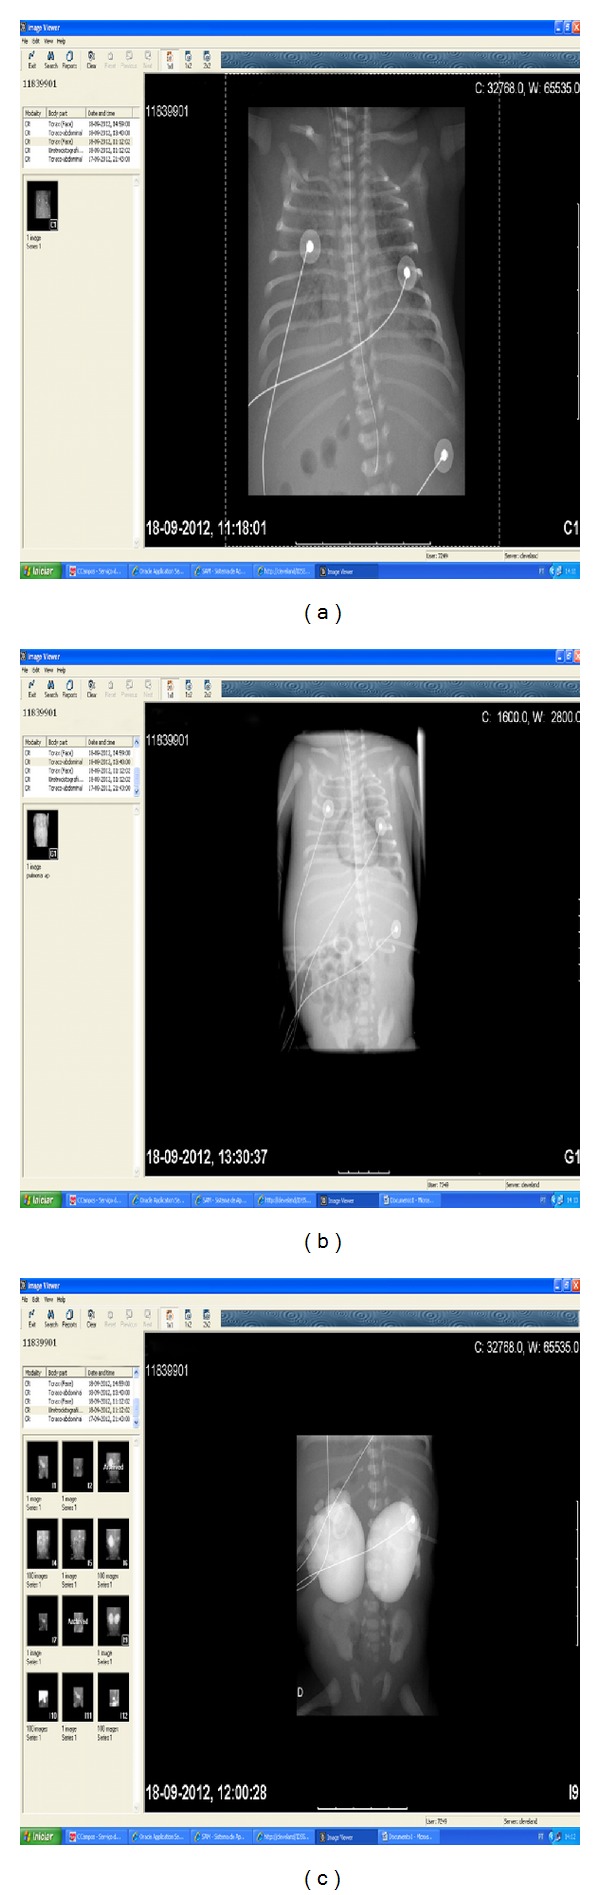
Case report 3: (a) chest radiograph with pulmonary ground glass opacities and right pleural effusion. (b) Chest radiograph showing pneumomediastinum. (c) Percutaneous nephrostomy using water-soluble contrast revealing very severe bilateral hydronephrosis.
